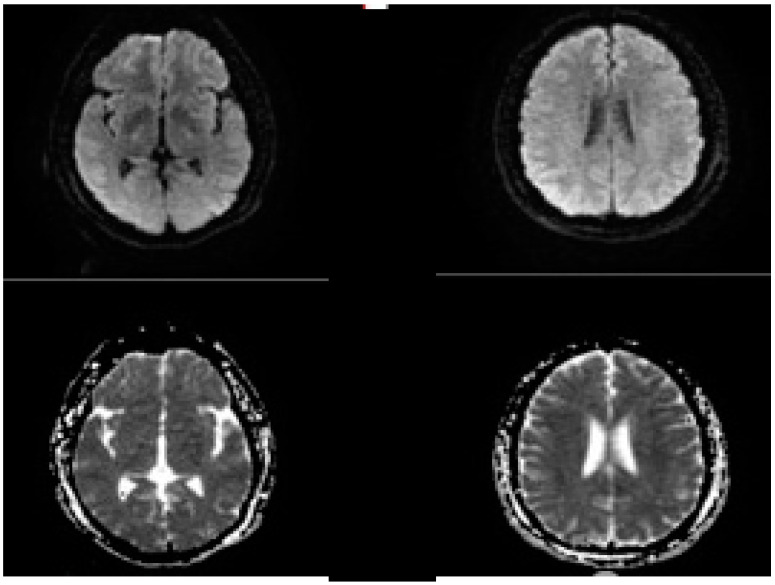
Post procedure of MRI brain in the same patient presenting with left MCA occlusion, no DWI lesions were seen.
